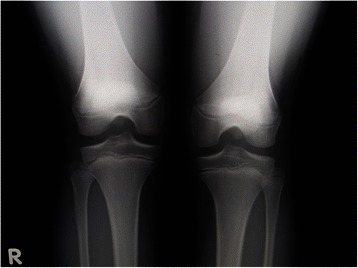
A posterior-anterior weight bearing radiograph (Rosenberg’s view) of the patient’s left knee at 3 years and 6 months after reoperation showing radiological healing of the osteochondritis dissecans lesions in both knees
